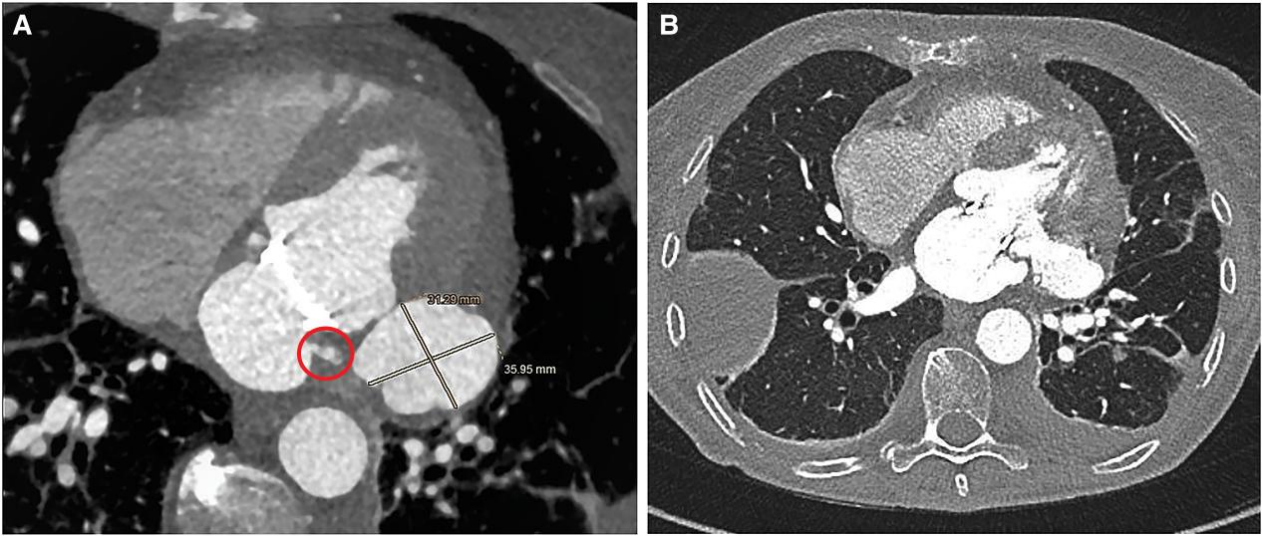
(*A*) and (*B*) Axial cardiac computed tomography angiography demonstrating the left ventricular pseudoaneurysm at the level of antero-lateral commissure.
